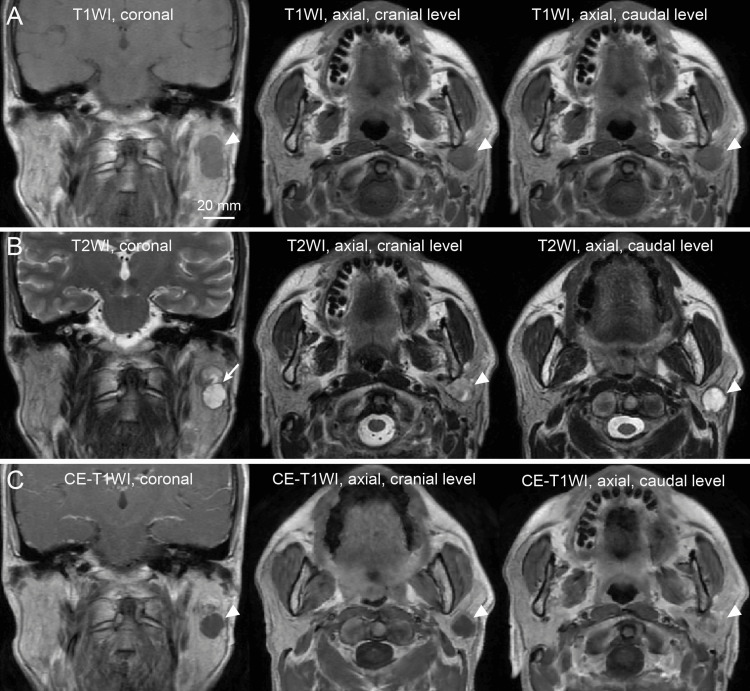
Magnetic resonance imaging (MRI) findings. (A) T1-weighted images (T1WI). Left: Coronal view. Center: Axial image at the level of the cranial component. Right: Axial image at the level of the caudal component. (B) T2-weighted images (T2WI). Left: Coronal view. Center: Axial image at the level of the cranial component. Right: Axial image at the level of the caudal component. The arrow indicates the capsule-like rim at the interface between the cranial and caudal tumor components, demonstrating structural compartmentalization. This interface represents a structural boundary suggestive of biologically distinct tumor components. (C) Contrast-enhanced T1-weighted images (CE-T1WI). Left: Coronal view. Center: Axial image at the level of the cranial component. Right: Axial image at the level of the caudal component. Arrowheads indicate the tumor in each panel.
